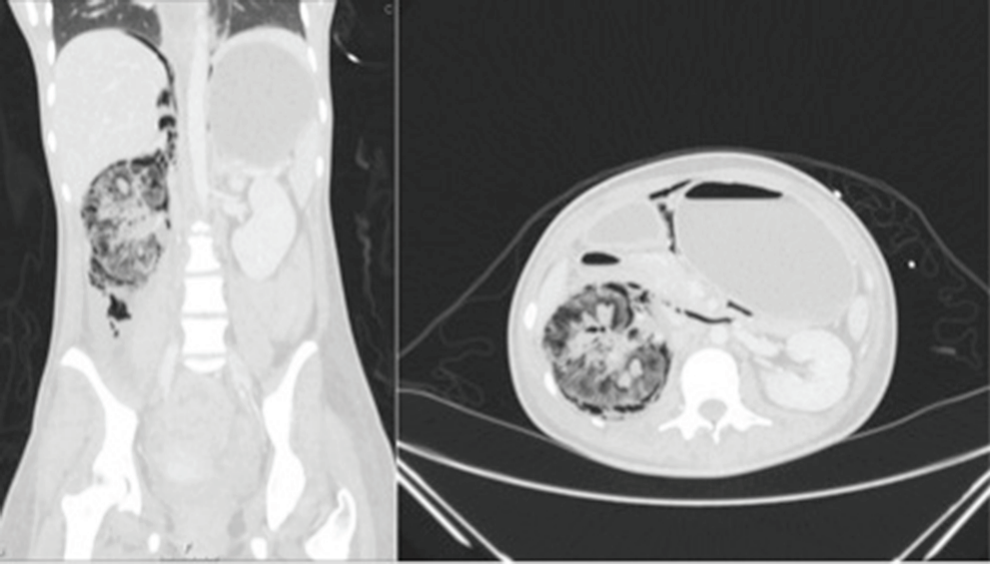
CT image of lung windows showing grossly emphysematous right kidney. Gas is also seen tracking into the retroperiotenum and subphrenic space.
